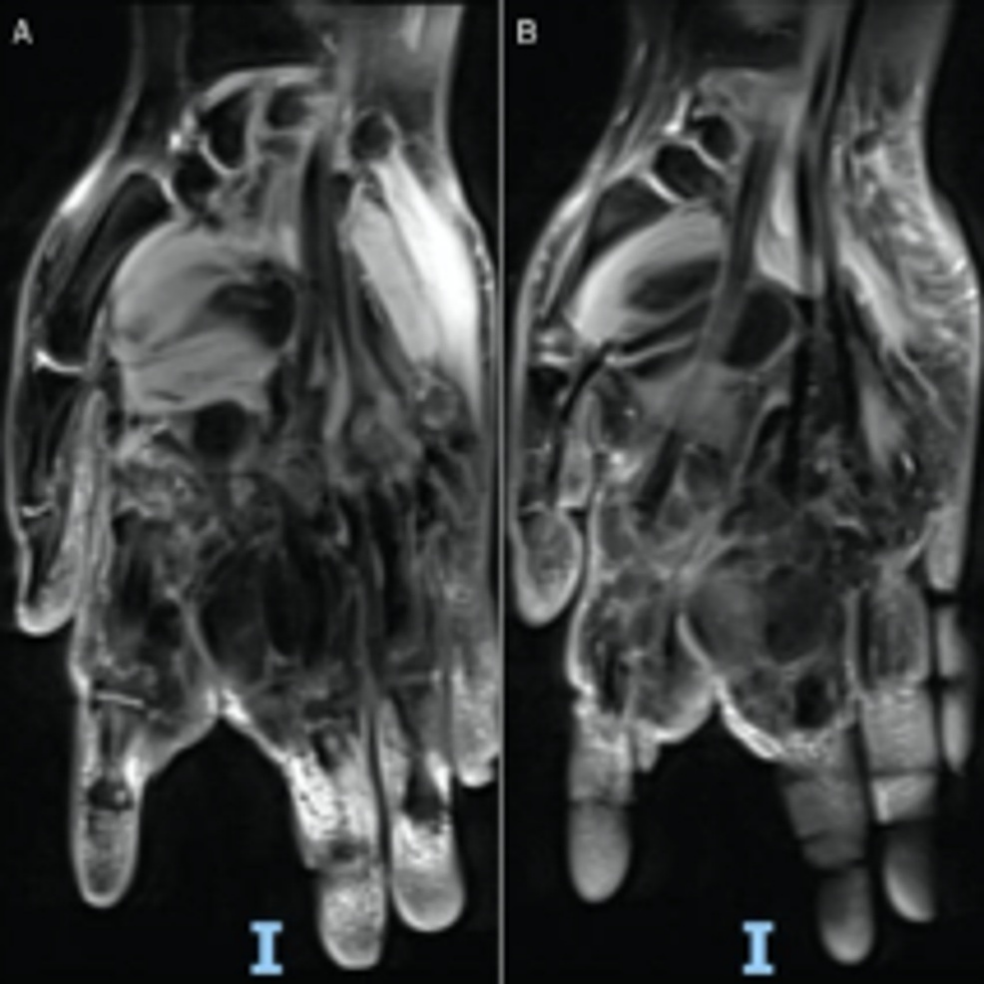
A and B are MRI-T1 images of the left hand showing the lipoma of the index and middle digits.
